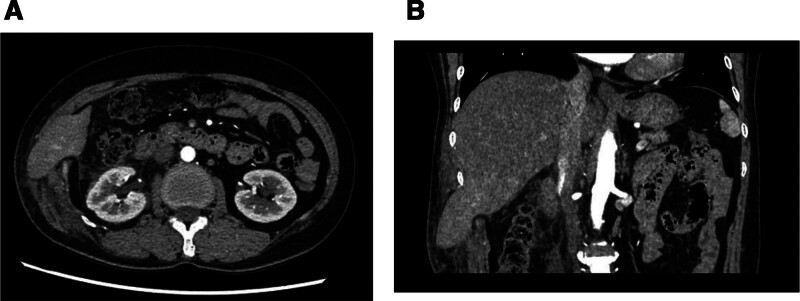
(A and B) CT revealed herniation of the anterolateral segment of the right hepatic lobe through a diaphragmatic defect, accompanied by significant soft tissue edema and fat stranding within the adjacent right abdominal wall. CT = computed tomography.
